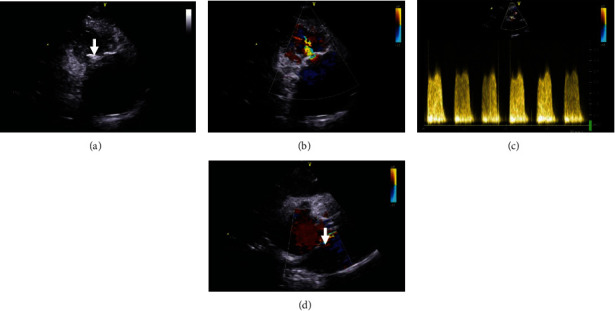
Transthoracic echocardiography (TTE) and Doppler before the operation: bioprosthetic tricuspid valve calcification (white arrow) and stenosis (a, b). Bioprosthetic maximal velocity 2.7 m/s (c). The foramen ovale opening (white arrow) (d).
